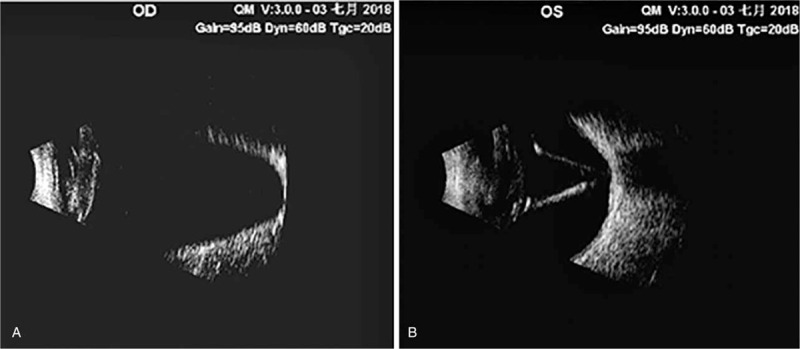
B-scan ultrasonography of both eyes. (A) The right eye showed hallmark posterior staphylomas. (B) The left eye showed retinal detachment and atrophy.
